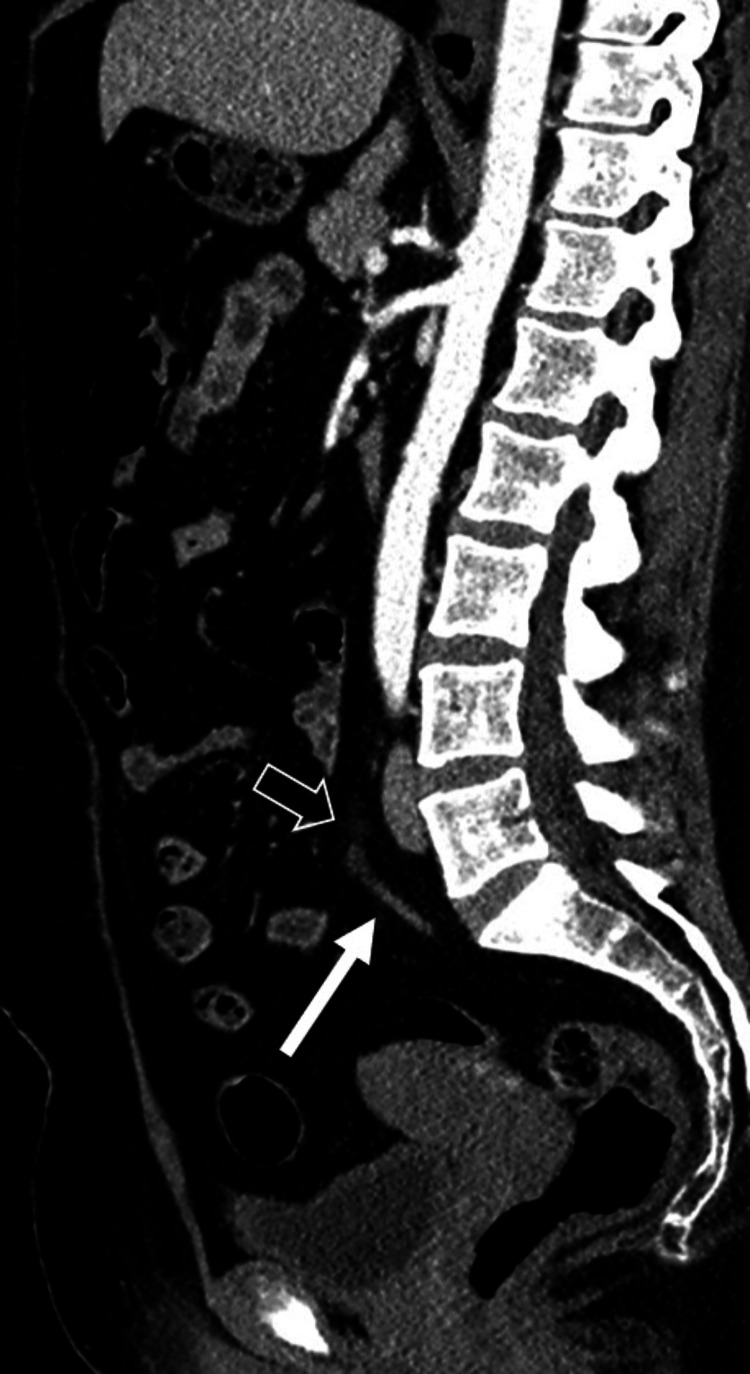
CT of the abdomen and pelvis with contrast (sagittal view) showing mild focal dilation of the appendiceal tip with subtle periappendiceal fat stranding (transparent white arrow). The rest of the appendix appears unremarkable (solid white arrow), further supporting the suspicion of early-stage tip appendicitis.
